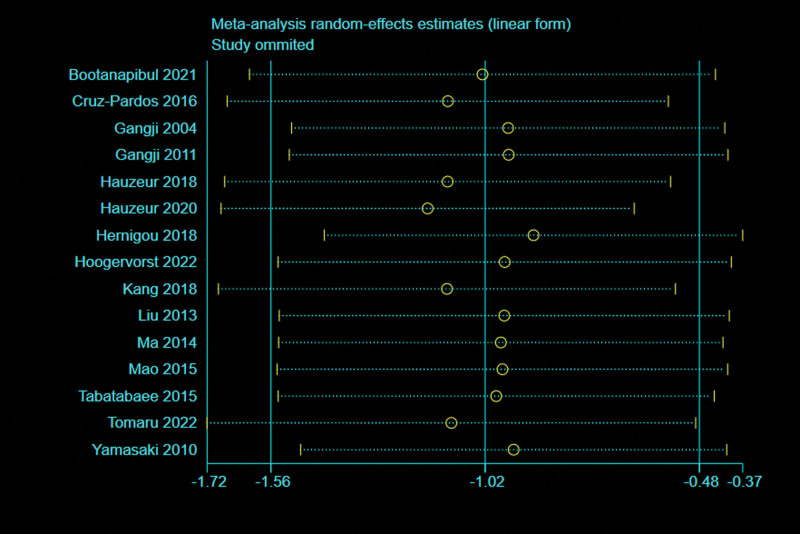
Sensitivity analysis of radiographic progression rate.
